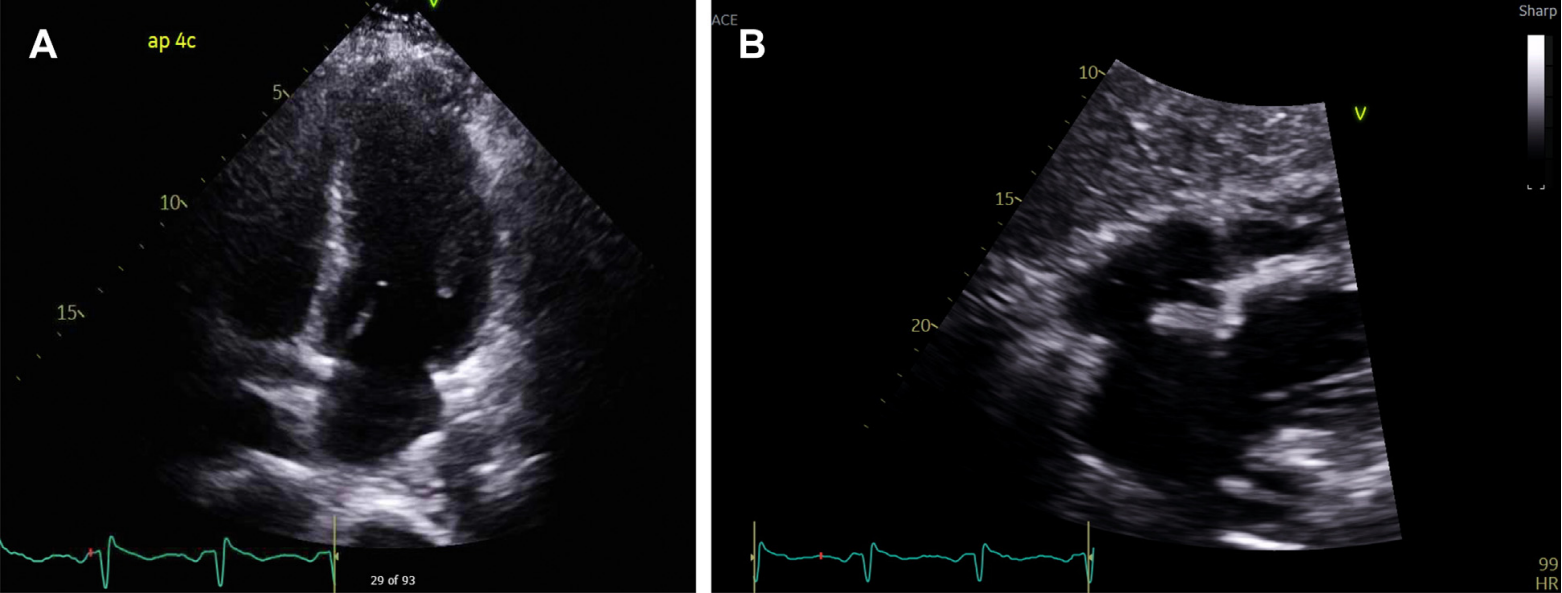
Initial Transthoracic Echocardiogram (A) Left ventricular ejection fraction of 45% with mild left ventricular global hypokinesis. (B) Subxiphoid pericardial view showing a normal left ventricular cavity size and a normal left atrium.
